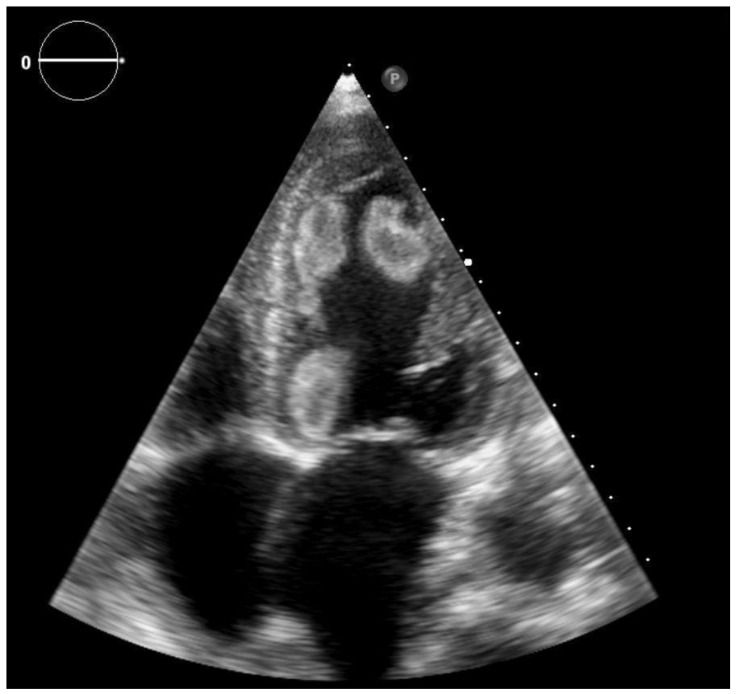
2D transthoracic echocardiography, apical four chamber view—the left ventricular cavity is filled by multiple thrombi: apical-septal (AS), -lateral (AL) and basal-infero-septal (BIS).
